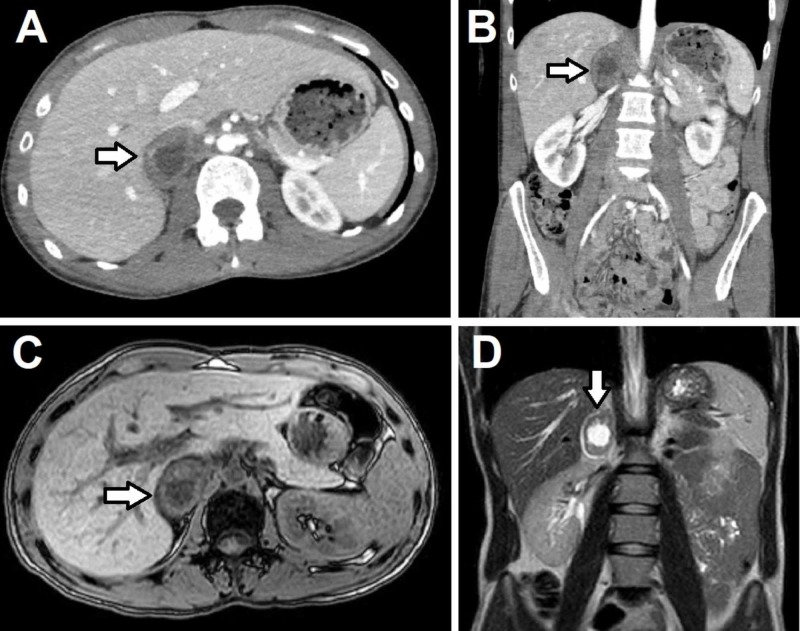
Computed tomography (CT) and magnetic resonance imaging (MRI) of the abdomen and pelvis (A) Axial and (B) coronal view of the CT scan of the abdomen and pelvis. (C) Axial view of the In and Out phase of the MRI of the abdomen and pelvis and (D) coronal view of the T2 phase of the MRI of the abdomen and pelvis. The arrows are pointing at a 3.7 cm x 2.9 cm x 4.3 cm heterogeneous mass in the right adrenal gland with central necrosis, irregular borders, calcifications and significant compression of the inferior vena cava.
